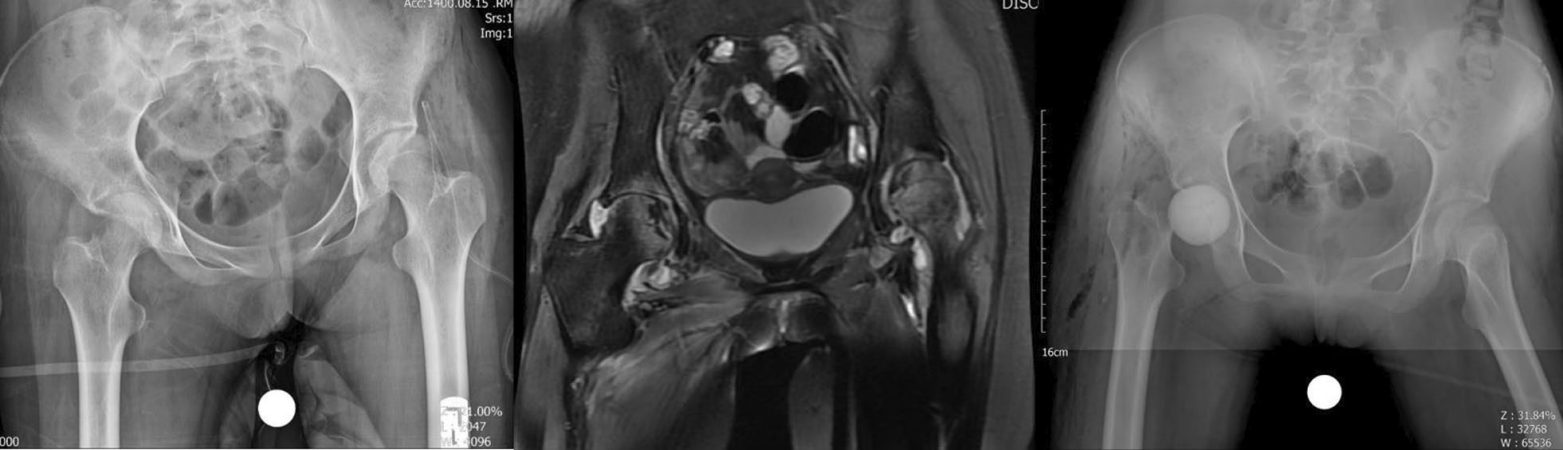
a Case two, preoperative X-ray, bilateral involvement. b MRI of both hips shows cartilage destruction on right side and acute inflammation on left side. c Post-op radiography after first stage of THA for right side and arthrotomy and irrigation and debridement for left side
